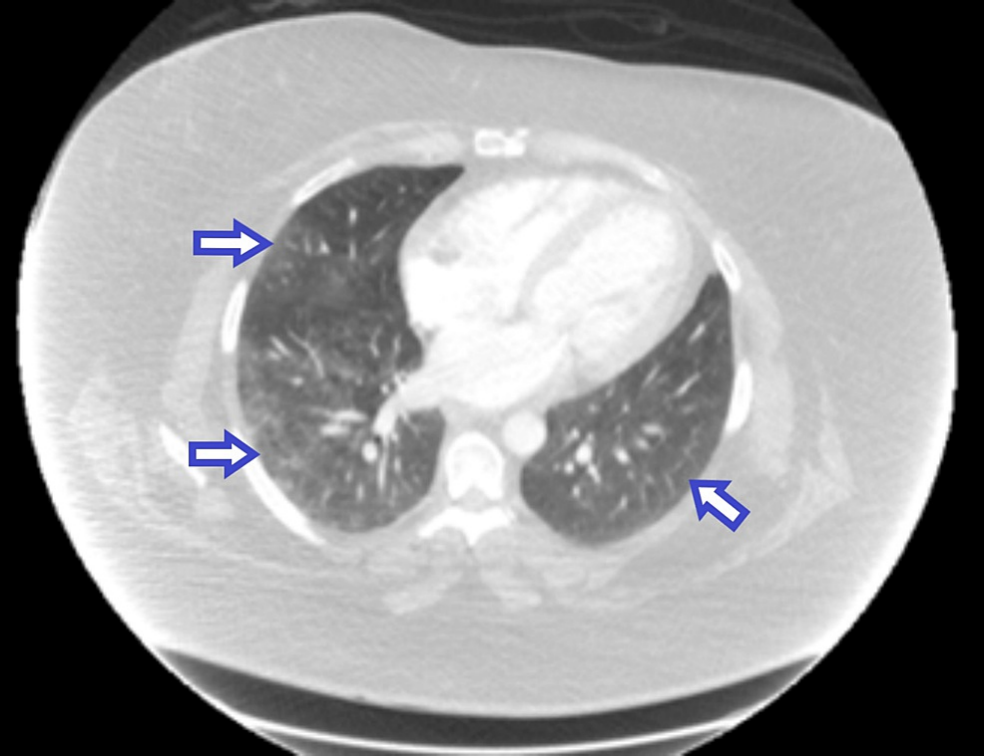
Chest CT scan with IV contrast showing bilateral ground glass opacities concerning pneumonitis as highlighted by the arrows
